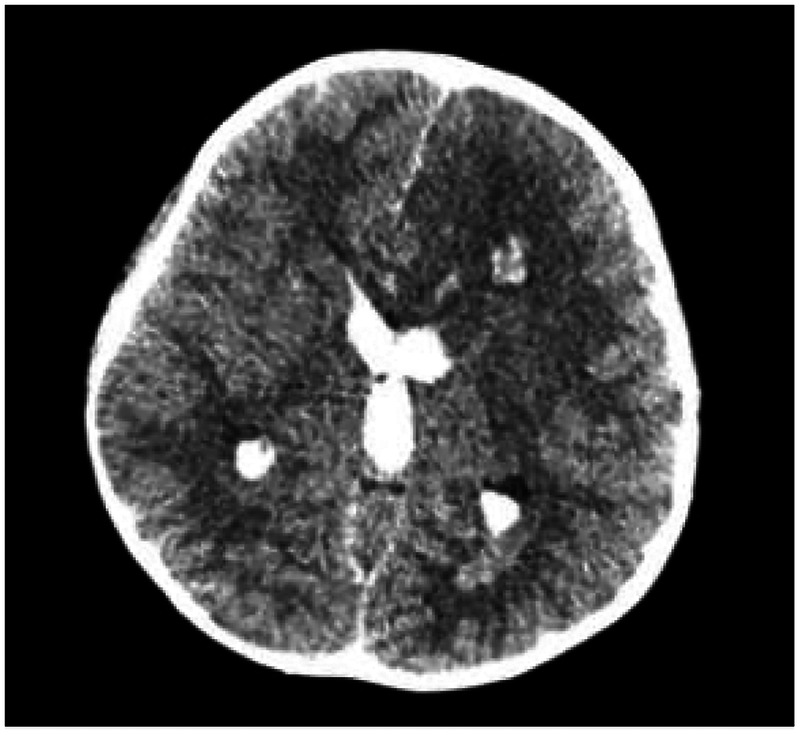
CT shows extensive intracerebral hemorrhage with marked midline shift of transtentorial herniation.
